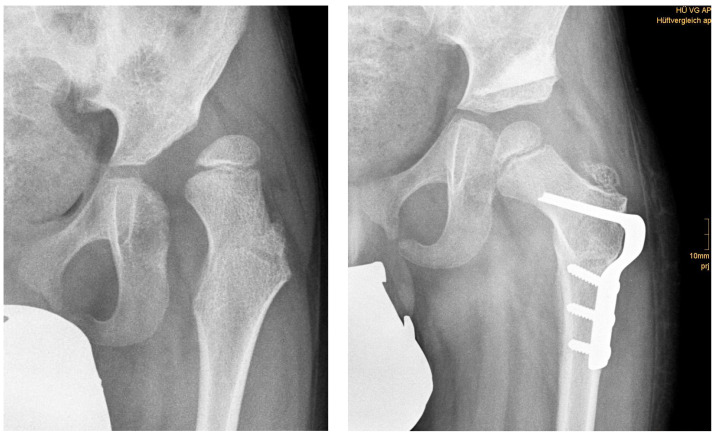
(left) Anteroposterior radiograph of a six-year-old male with NDH; (right) result 3 months postoperatively.
